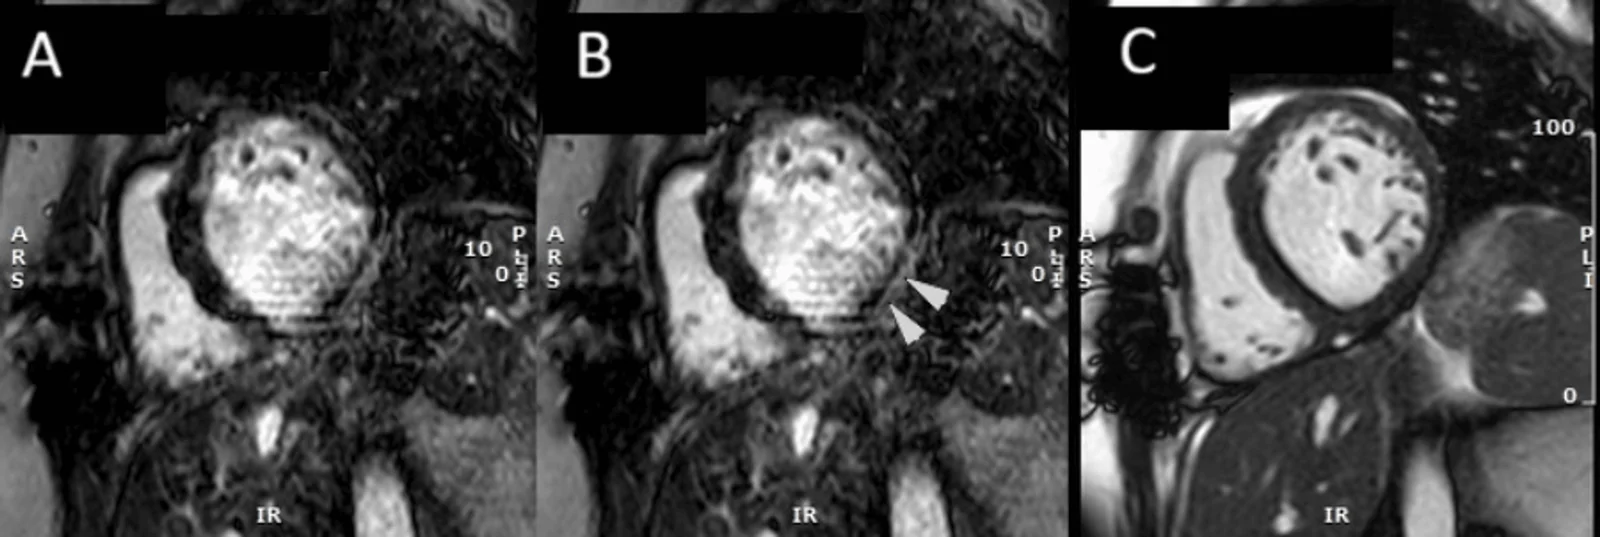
Short-axis cardiac MRI. (A-B) Delayed gadolinium enhancement images demonstrating mid-wall/subepicardial enhancement (arrows) supportive of nonischaemic myocardial injury consistent with myocarditis. (C) Matching short-axis cine image, shown to confirm this signal represents true late gadolinium enhancement rather than subepicardial fat. Image artifact is present due to difficulty with breath-holding.
